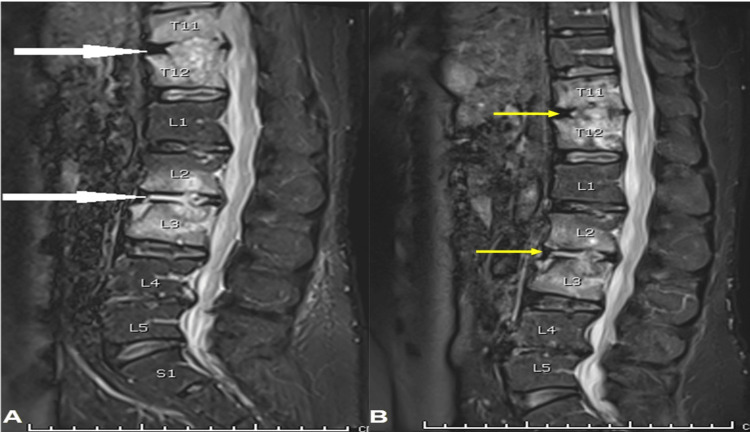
Sagittal MRI showing the inflammatory process at presentation and after four weeks (A) Sagittal MRI at presentation showing the large inflammatory process at the T11-T12 and L2-3 disc levels (white arrows). (B) Sagittal MRI after four weeks; inflammatory collections within the discs T11/T12 and L2/L3 levels still seen but decreased in size (yellow arrows).
